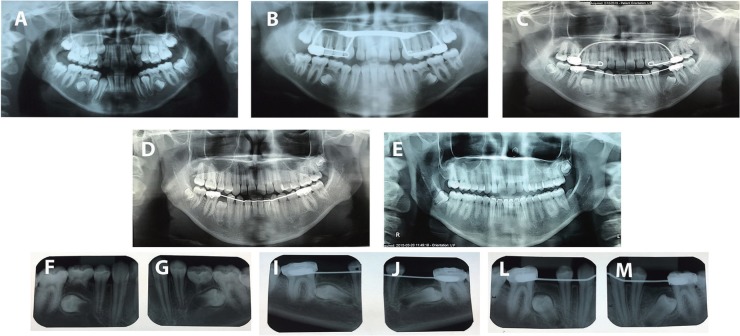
Panoramic and periapical radiographies monitoring of premolar eruption. A: Mandibular second premolars starting to turn backward. B: Mandibular second premolar 180º turned backward. C: Spontaneous uprighting, with mandibular lingual arch maintaining the E space. D: Eruption of mandibular second premolars. E: Final result with proper root parallelism. E-G: Mandibular second premolars 180º turned backward. I-J: Extraction of the mandibular primary second molars. L-M: Spontaneous uprighting of the mandibular second premolars.
